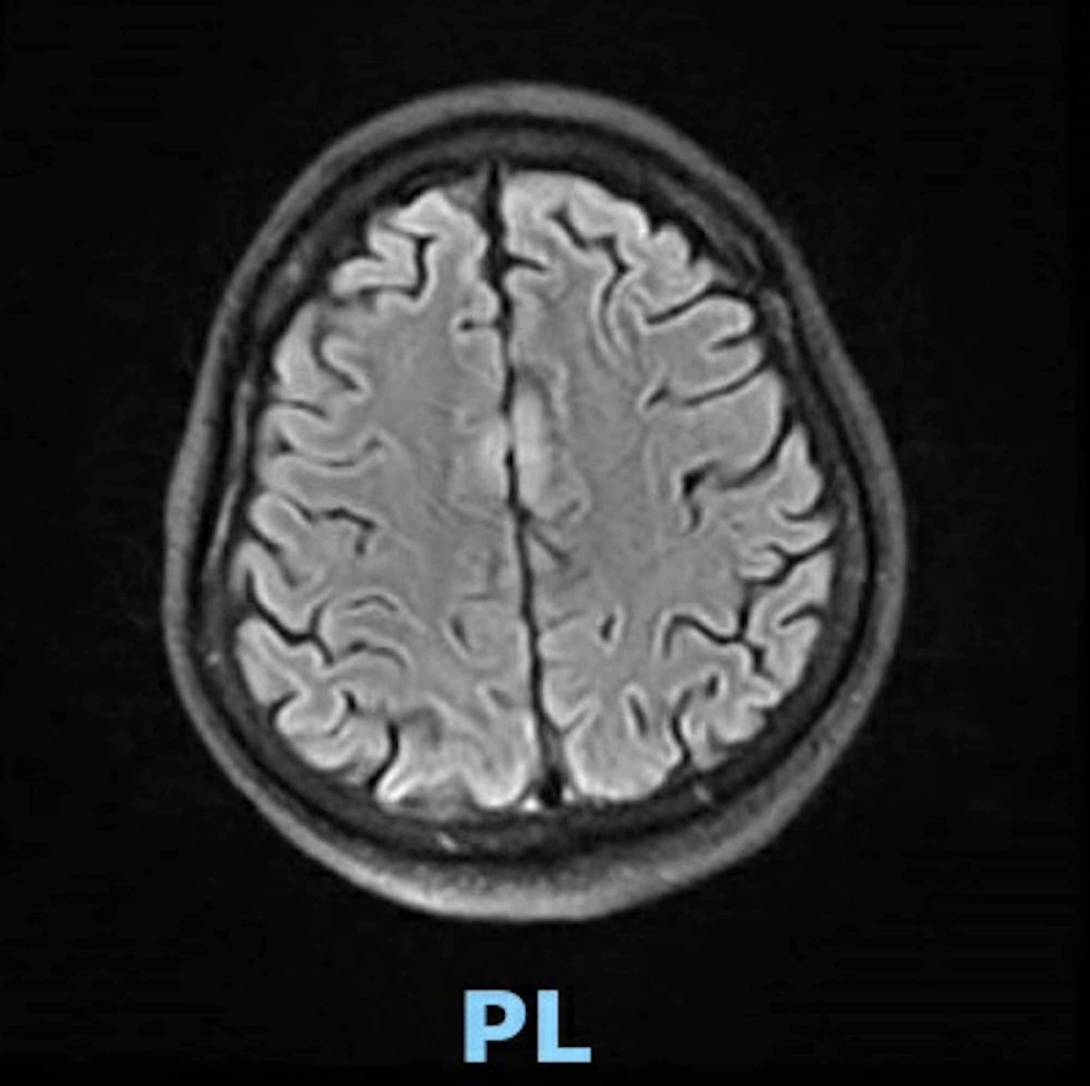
MRI brain showing abnormal medial cortical signals in the bilateral frontal lobes MRI: magnetic resonance imaging
